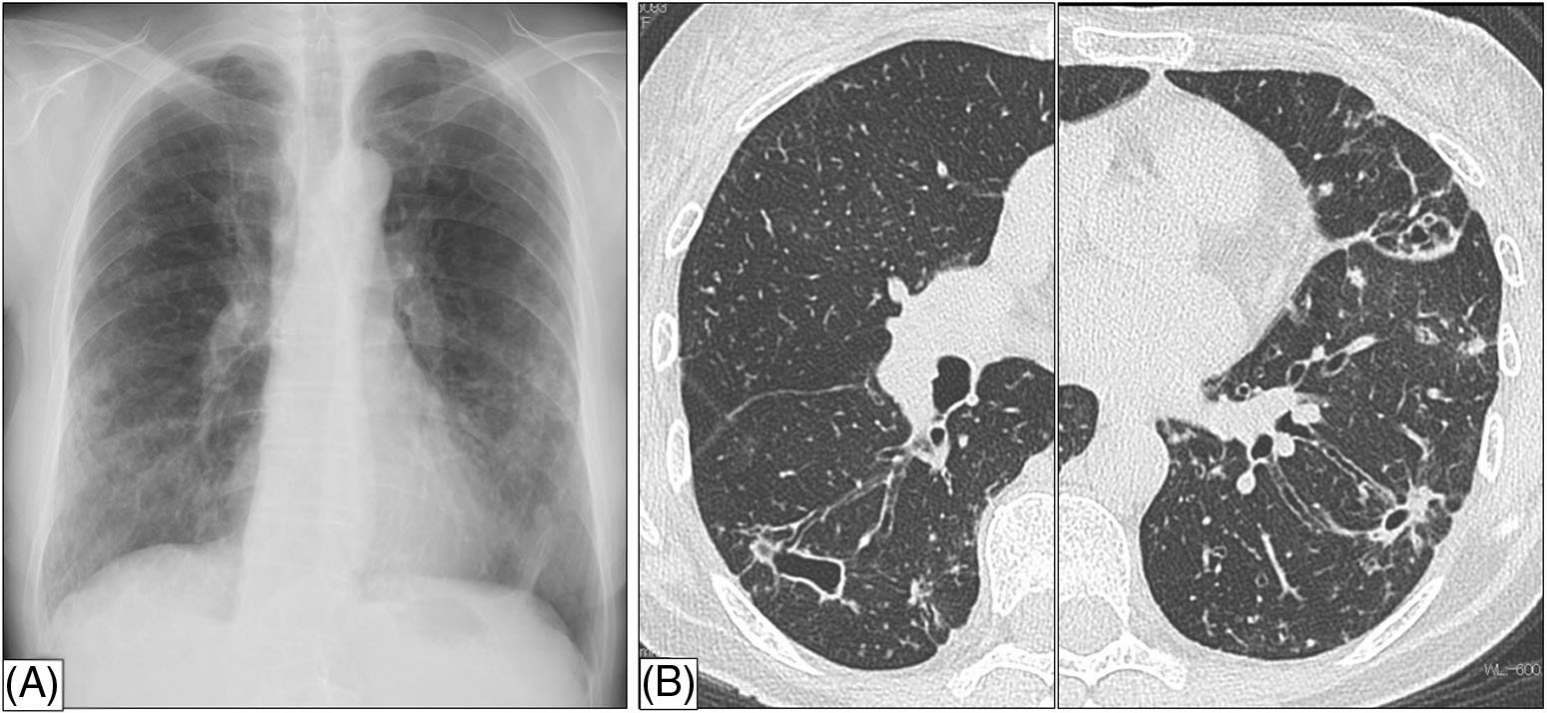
(A) Chest radiograph showing predominantly peripheral opacities and small nodules, bilaterally. (B) High‐resolution CT showing bilateral bronchiectasis, centrilobular nodules, and cavities
